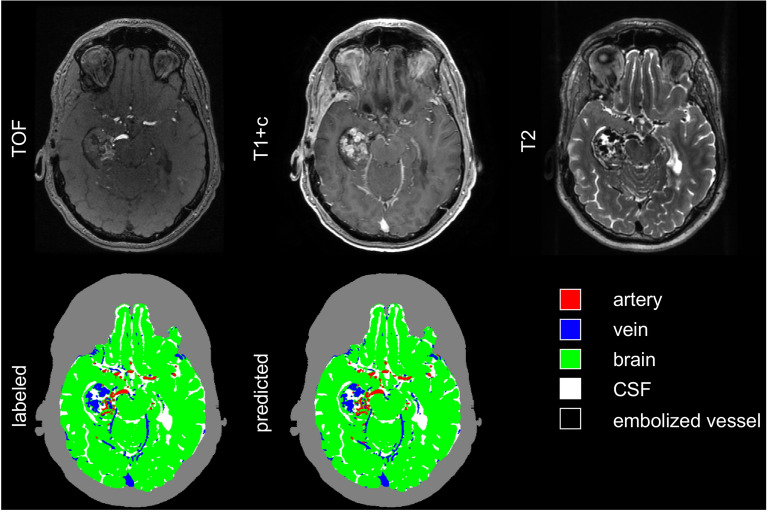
Representative images of an AVM nidus in the right temporal lobe as demonstrated by multi-modal MRI/MRA and vascular anatomical maps. Dice similarity coefficients for this slice: arteries 0.85; veins 0.84; brain 0.98; CSF 0.94; embolized vessels 0 (not present). The grey contour outlining the extracranial space is not generated by the segmentation algorithm and is included for visual reference only. AVM: arteriovenous malformation, MRI: magnetic resonance imaging, MRA: magnetic resonance angiography, CSF: cerebral spinal fluid.
